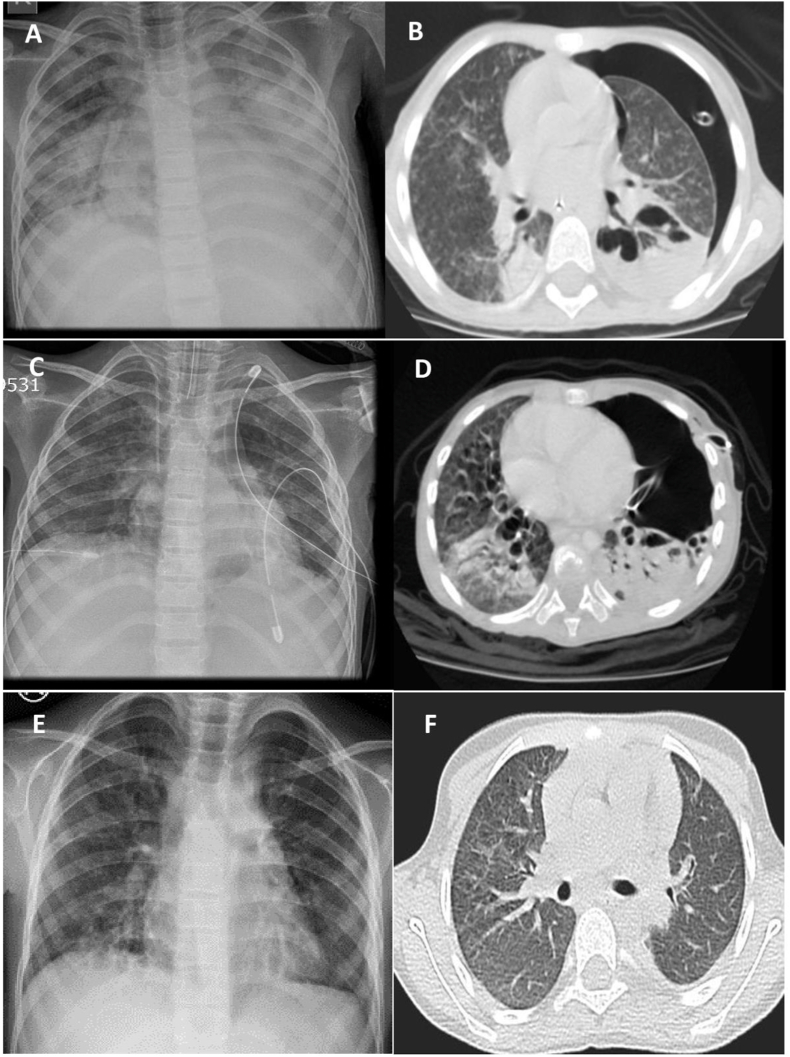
(A) Chest xray showing left side pneumothorax. (B&C) Chest ray &CT scan showed chest drain inserted. (D) Recurent pneumothorax after surgery. (E&F) After closure of Bronchpleural fistula.
